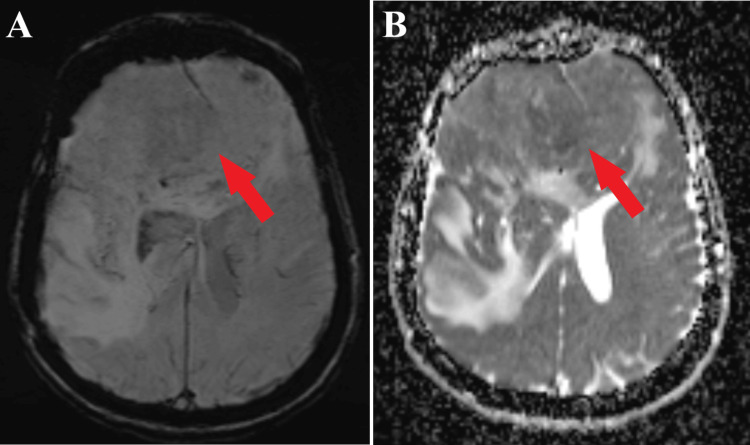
Preoperative MRI SWI and ADC sequence, axial section. A: MRI SWI sequence highlights the giant meningioma (red arrow).
